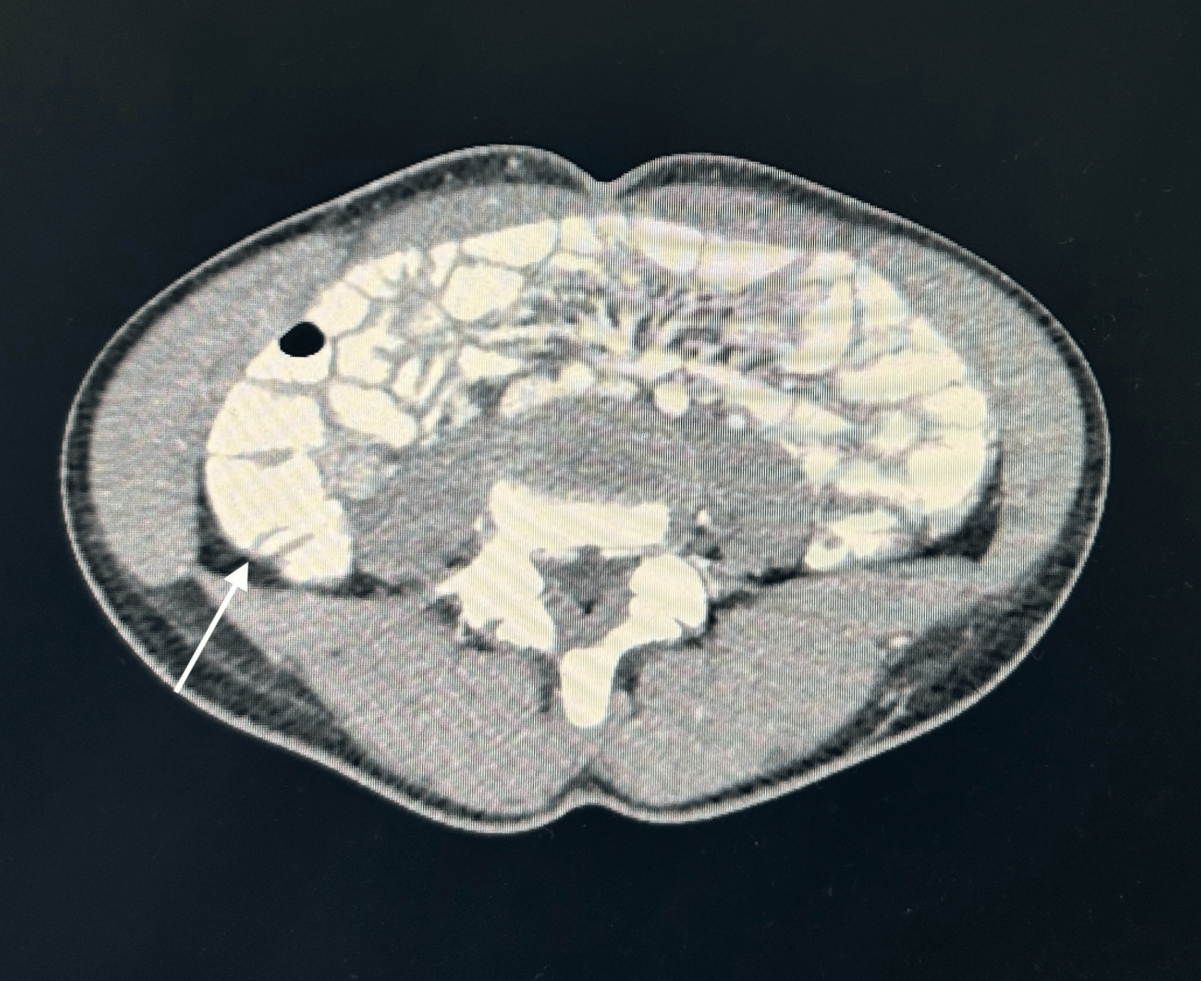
CT axial view with normal appendix CT: computed tomography
